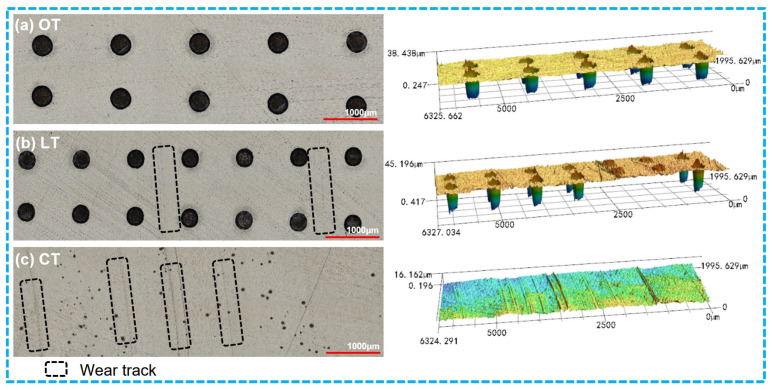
Representative worn surfaces and 3D morphologies of the 40# steel discs of OT, LT and CT after ultrasonic cleaning. (a) OT; (b) LT; (c) CT.
